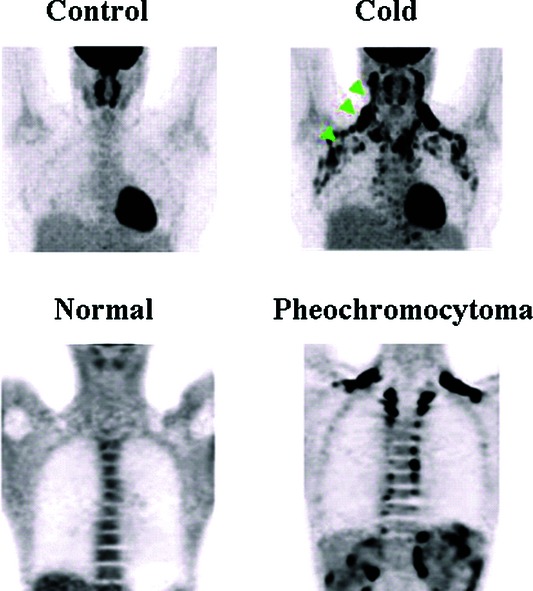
BAT activities in humans Top panels, BAT activities measured by FDG-PET in a normal subject and a subject exposed to cold (from Ref. 48). Bottom panels, BAT activities in a normal subject and a pheochromocytoma patient with elevated circulating level of catecholamines (with permission from Ref. 57).
